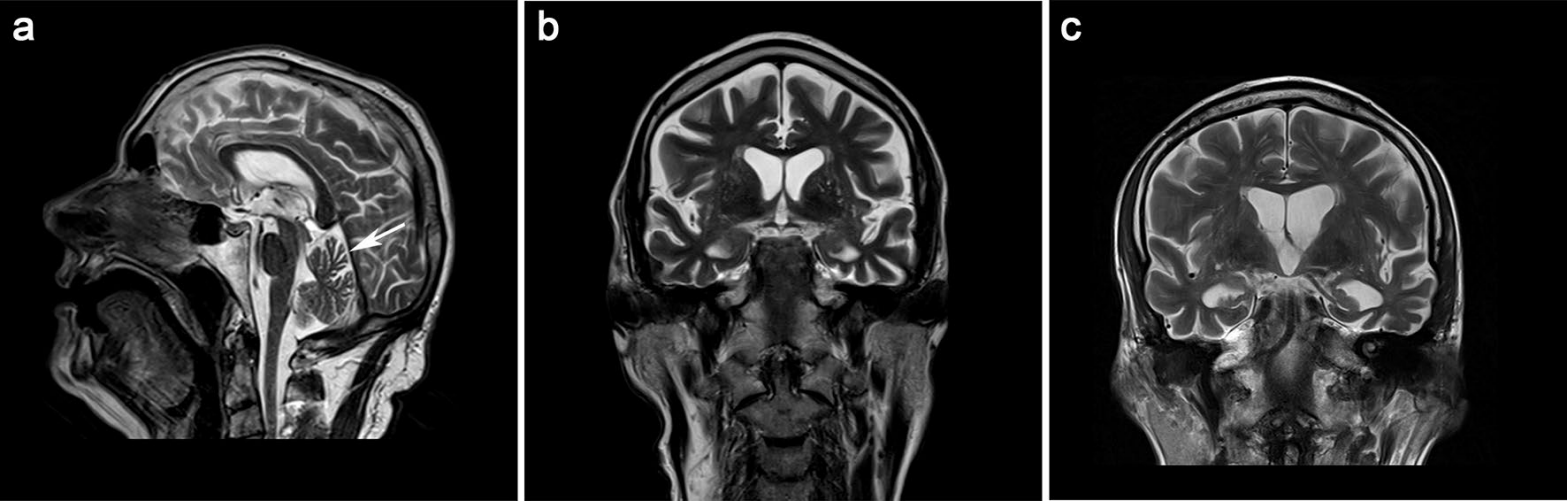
HSP- or FTD-associated patterns on brain MR images of ALS patients carrying heterozygous *SPG7* mutations. a Sagittal T2-weighted scan of patient VALS008 carrying the p.(R400W) variant demonstrates vermis atrophy (designated by an arrow). In addition, CC thinning was observed with a total CC area of 496 mm^2^. b, c Pronounced parietotemporal atrophy was detected in three of seven ALS patients carrying rare heterozygous *SPG7* variants, whereby coronal T2-weighted images of patient VALS008 carrying the p.(R400W) variant (b), and patient VALS125 carrying the p.(R486Q) variant (c) are shown
